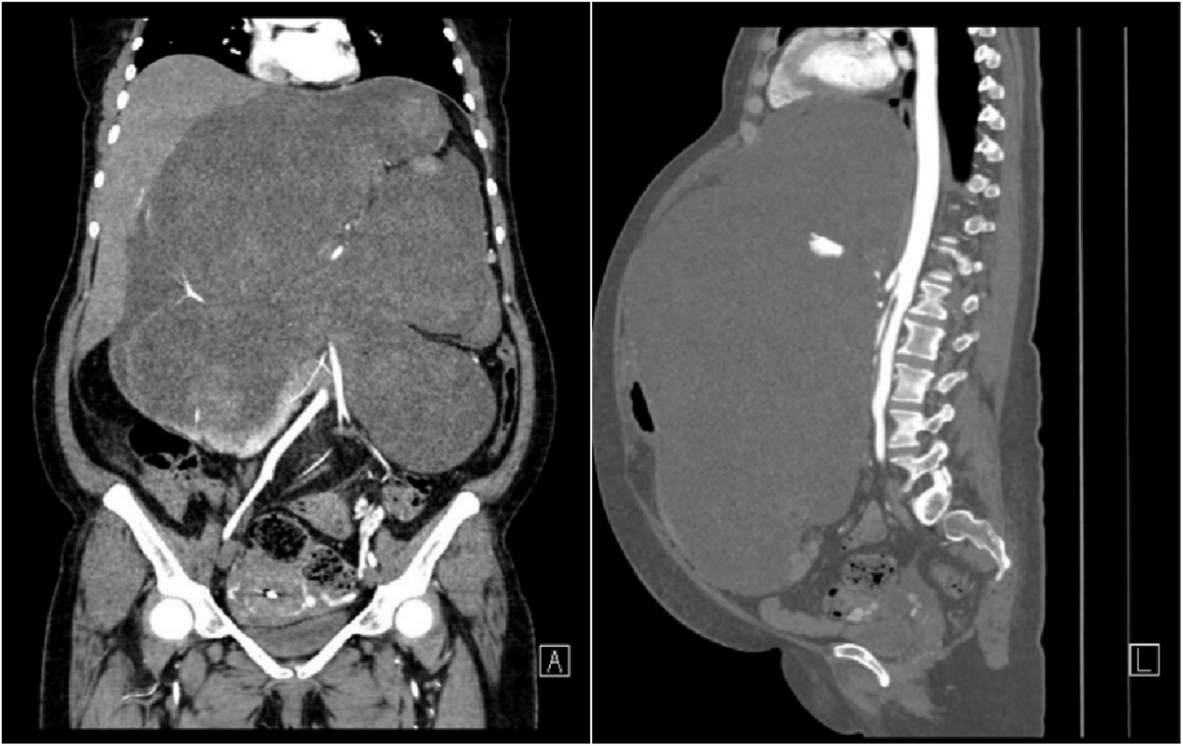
Three-dimensional reconstructed CT images of the huge retroperitoneal tumor with a mass effect on the bowel. In the arterial phase, the tumor demonstrated uneven and mild enhancement. Anterior (A). Left side (L)
